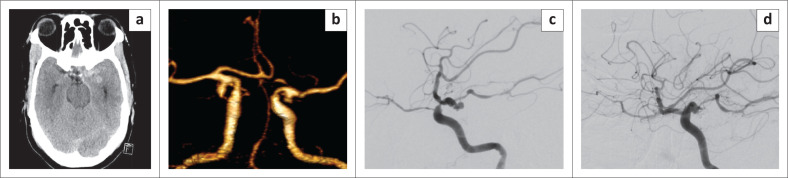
A 65-year old man presented with sudden onset of severe headache. Non-contrast computed tomography brain: (a) showed diffuse subarachnoid haemorrhage (SAH) with a heavier blood load on the left. Computed tomography angiogram (b) showed bilateral common clinical presentation of posterior communicating artery aneurysms. Digital substraction angiography (DSA) of the left internal carotid artery in the lateral projection shows the multilobulated posteriorly directed aneurysm before (c) and after (d) simple coiling embolisation.
